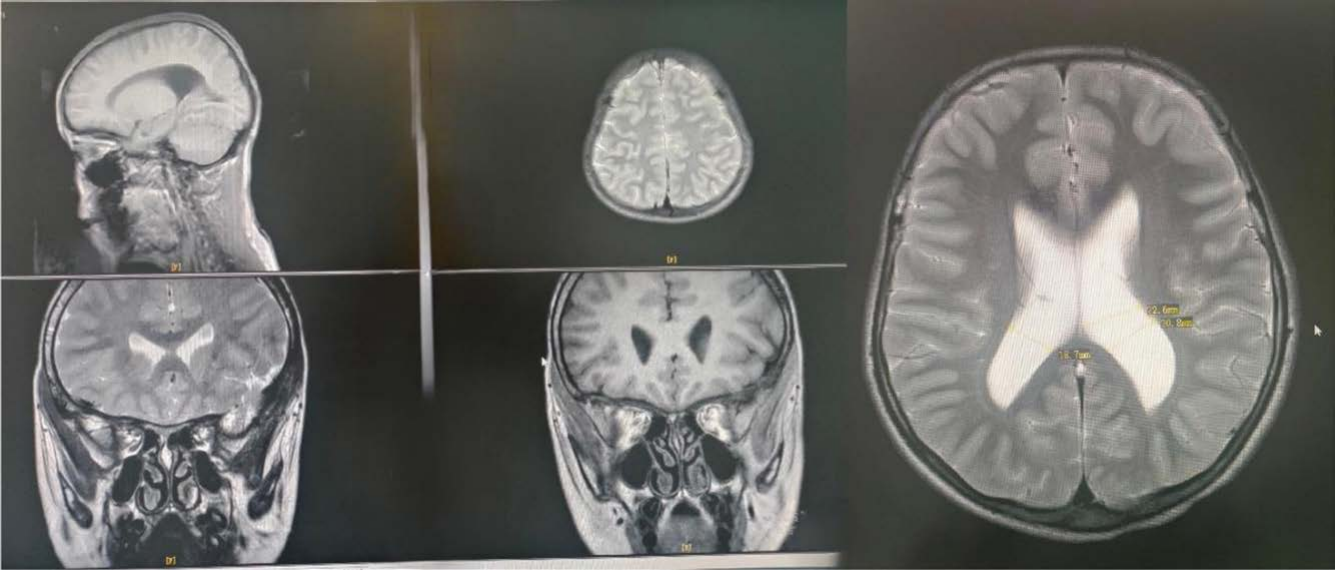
Magnetic resonance imaging of the brain revealed widening of bilateral lateral ventricles (left ventricle 2 cm and right ventricle 1.8 cm).
